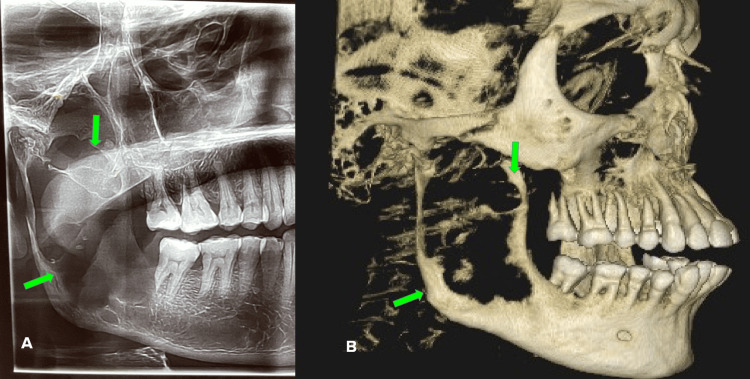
Radiograph revealing diffuse radiolucency involving the right mandibular ramus extending into the condyle along with perforation of cortical plates as seen in the orthopantomogram (A) and cone beam computed tomography (CBCT) (B) Green arrows indicate the osteolytic lesion of the right mandible.
